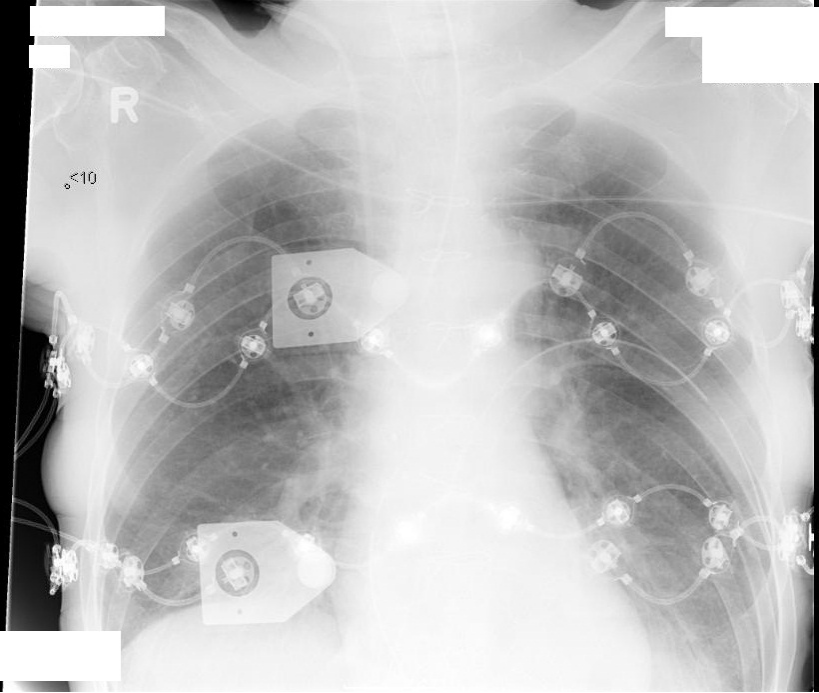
Chest X-ray of one of the studied patients showing the cranial (upper) and caudal (lower) electrical impedance tomography belt. X-ray taken at 5 cm H_2_O positive end-expiratory pressure (PEEP).
